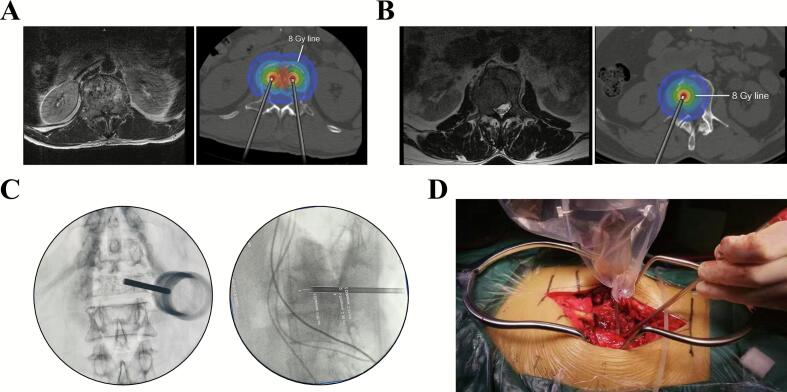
Procedure of MSS-IORT. (A) Preoperative planning for IORT for a tumor invading the bilateral pedicles, lamina, and entire vertebral body. (B) Preoperative planning for IORT for a tumor invading the unilateral pedicle, lamina, and part of the vertebral body. (C) The C-arm X-ray machine captured anteroposterior and lateral fluoroscopy of the applicator during the operation. (D) Intraoperative image of the IORT.
